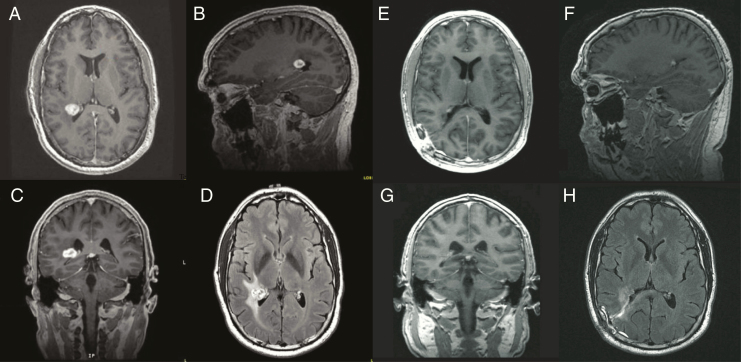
(A–D) Preoperative magnetic resonance imaging (MRI). Enhancing lesion in the lateral ependymal surface of the right lateral trigone measuring 1.7 × 1.4 × 1.2 cm. The medial border is intimately associated with the adjacent choroid plexus. Surrounding vasogenic edema in the periventricular white matter of the right parietal lobe. (A–C) T1-weighted imaging post-gadolinium contrast representative axial, sagittal, and coronal images, respectively. (D) Representative axial T2 FLAIR image demonstrating perilesional edema. (E–H) Postoperative MRI following right parietal craniotomy for tumor resection. A small amount of residual postoperative fluid collection and associated dural thickening adjacent to the craniotomy site shown with the surgical tract leading toward the atrium of the right lateral ventricle. (E–G) Representative axial, sagittal, and coronal T1-weighted imaging post-gadolinium contrast images, respectively. (H) T2 FLAIR image demonstrating a decrease in perilesional edema compared to preoperatively.
